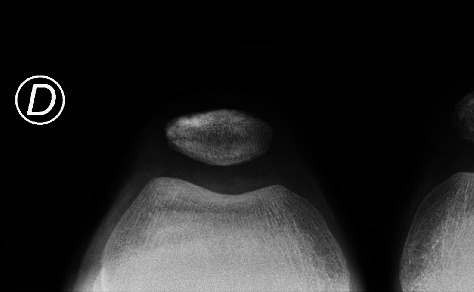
X-ray 3. Axial view of both knees (right knee on the left), initial radiological findings.
